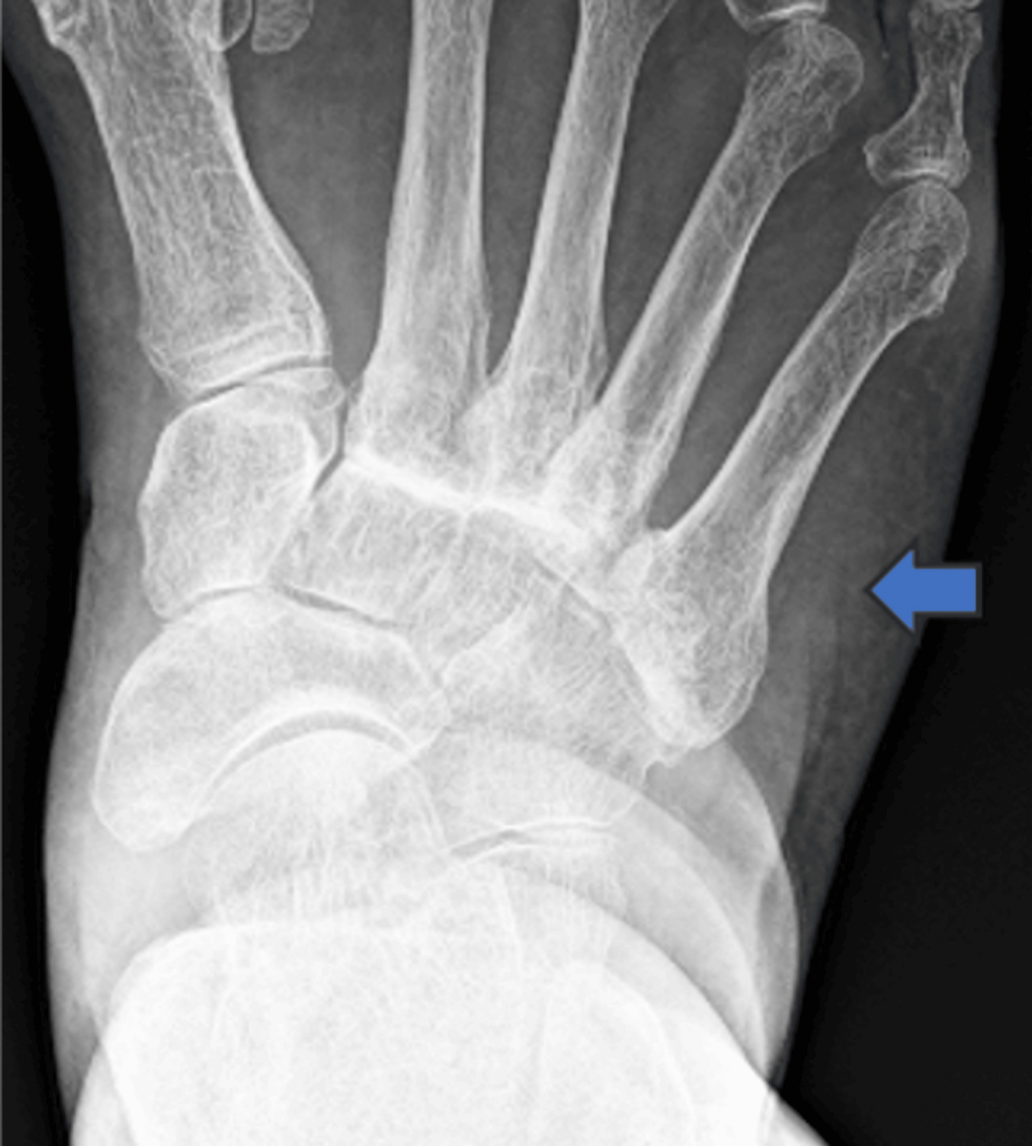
Preoperative X-ray The arrow indicates degenerative changes in the TMT joints, including hardening of the joint lines. TMT: tarsometatarsal
